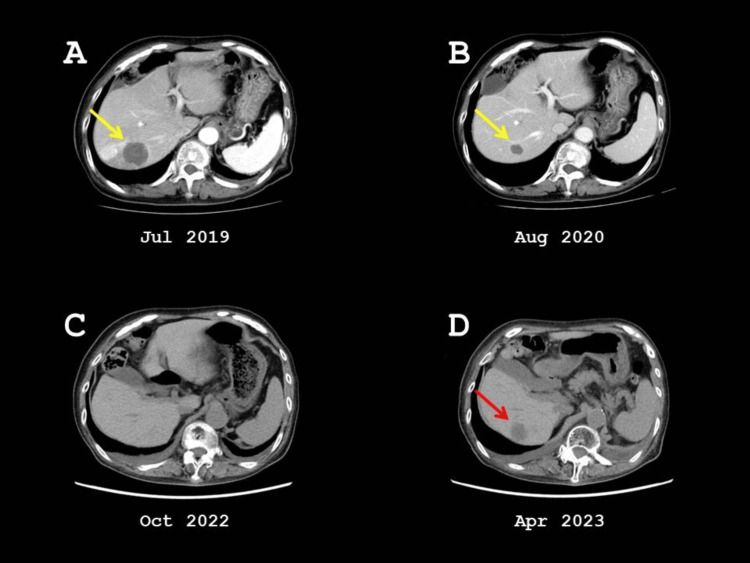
CT images after RFA. (A, B) The necrotic hepatic metastasis in segment 7 (yellow arrow) gradually decreased in size following RFA and eventually disappeared. (C) No new hepatic metastasis was observed until October 2022. (D) A new hepatic metastasis in segment 6 (red arrow) was detected in April 2023. (A) and (B) are enhanced CT scans; (C) and (D) are plain CT scans.
